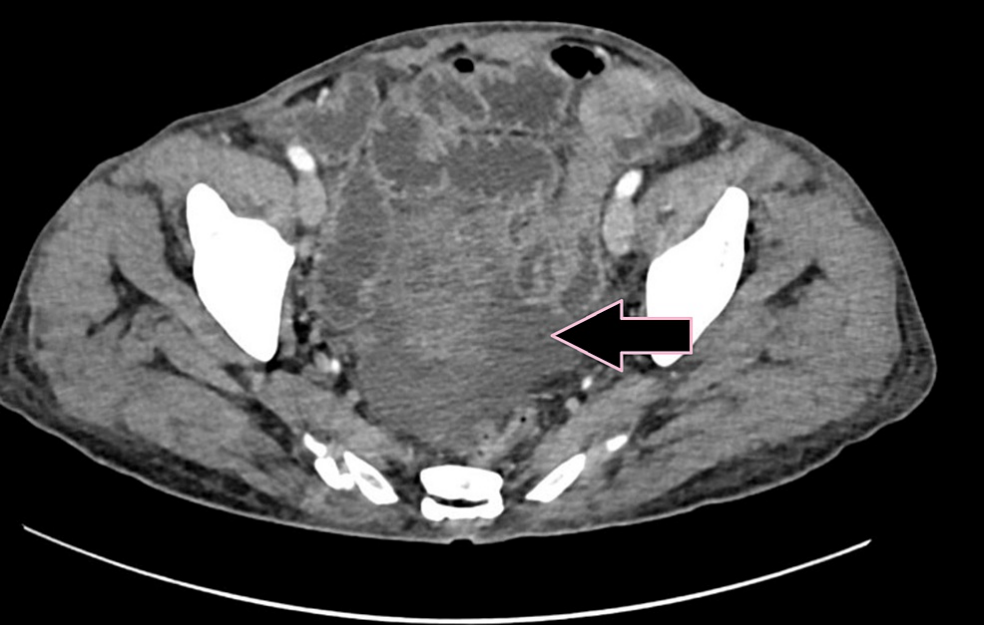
Axial DECT image showing free fluid in peritoneal cavity—ascites (pink arrow) (case 2). DECT: dual-energy computed tomography
